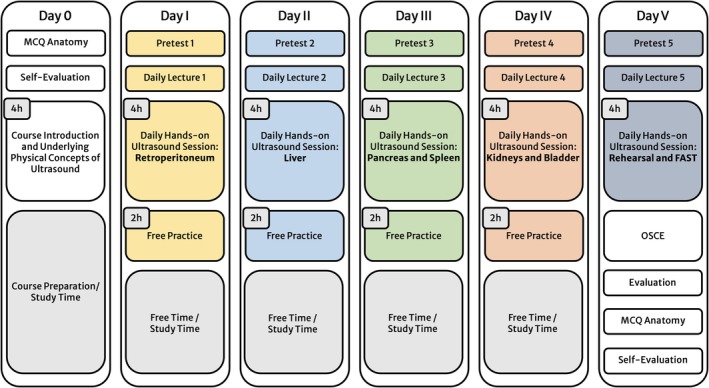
Course concept and data collection. FAST, focused assessment with sonography in trauma, MCQ, multiple‐choice question; OSCE, objective structured clinical examination.
